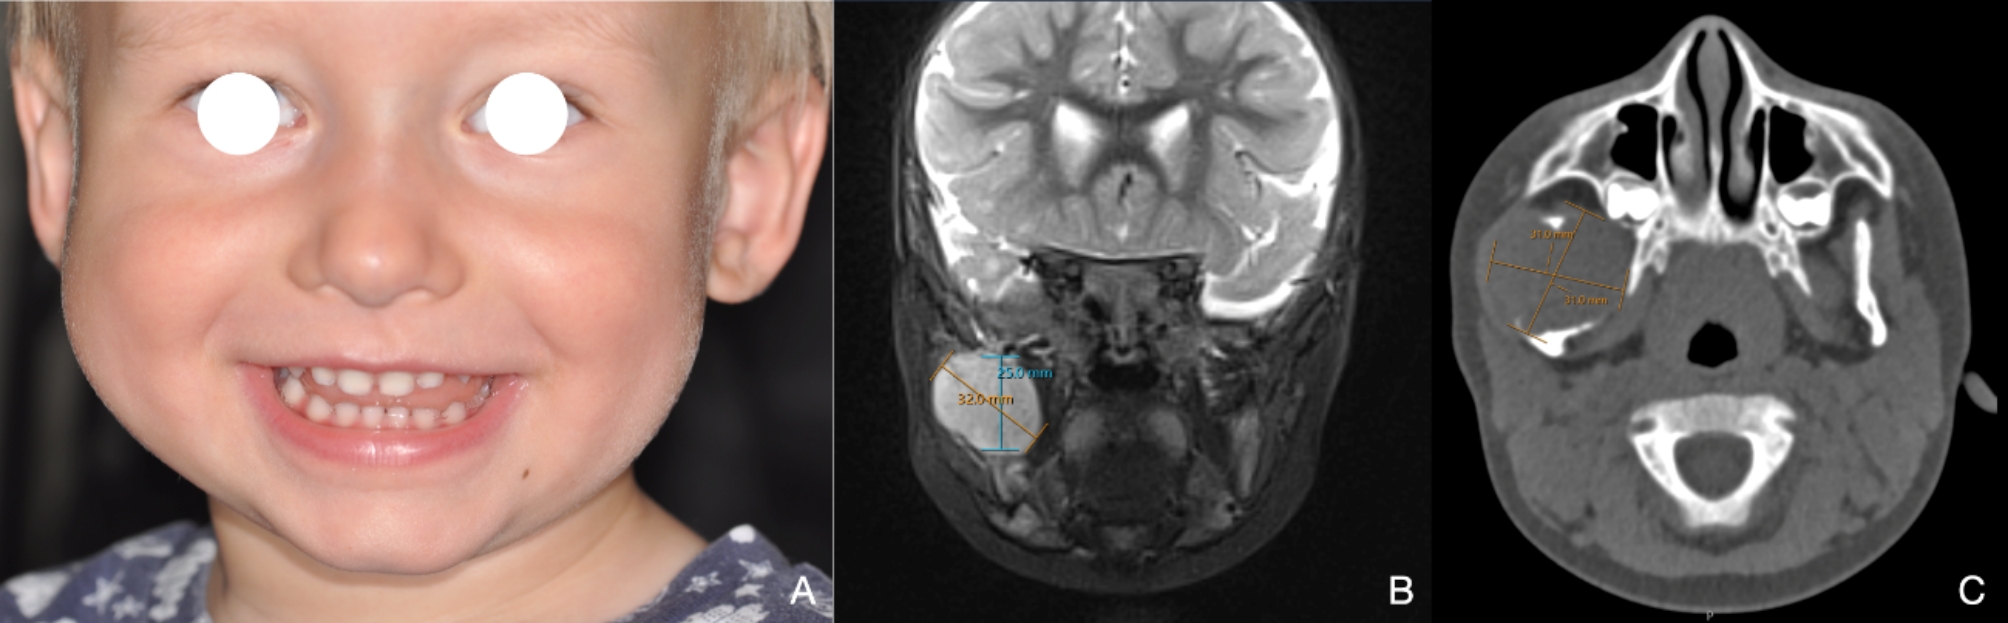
A, Clinical frontal view at consultation with volume plus on the right side. B, MRI showing a well-delineated intraosseous contrast-enhancing mass along the right mandibular ramus and coronoid process without signs of infiltrative growth or neck involvement. C, CT highlighting the lesion at the full height of the coronoid process, extending medially into the pterygomaxillary and laterally into the masticator space
